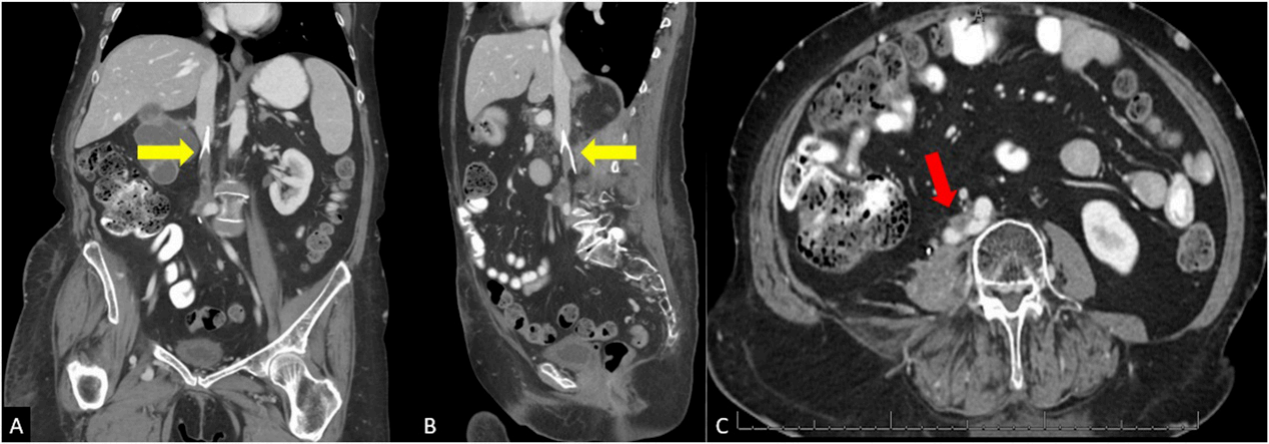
(A) Coronal and sagittal (B) CT shows chronic occlusion of the IVC with pre-existing filter (yellow arrow). (B) Axial images slightly lower show compression of the right common iliac by a mass prior to venous stenting (red arrow).
